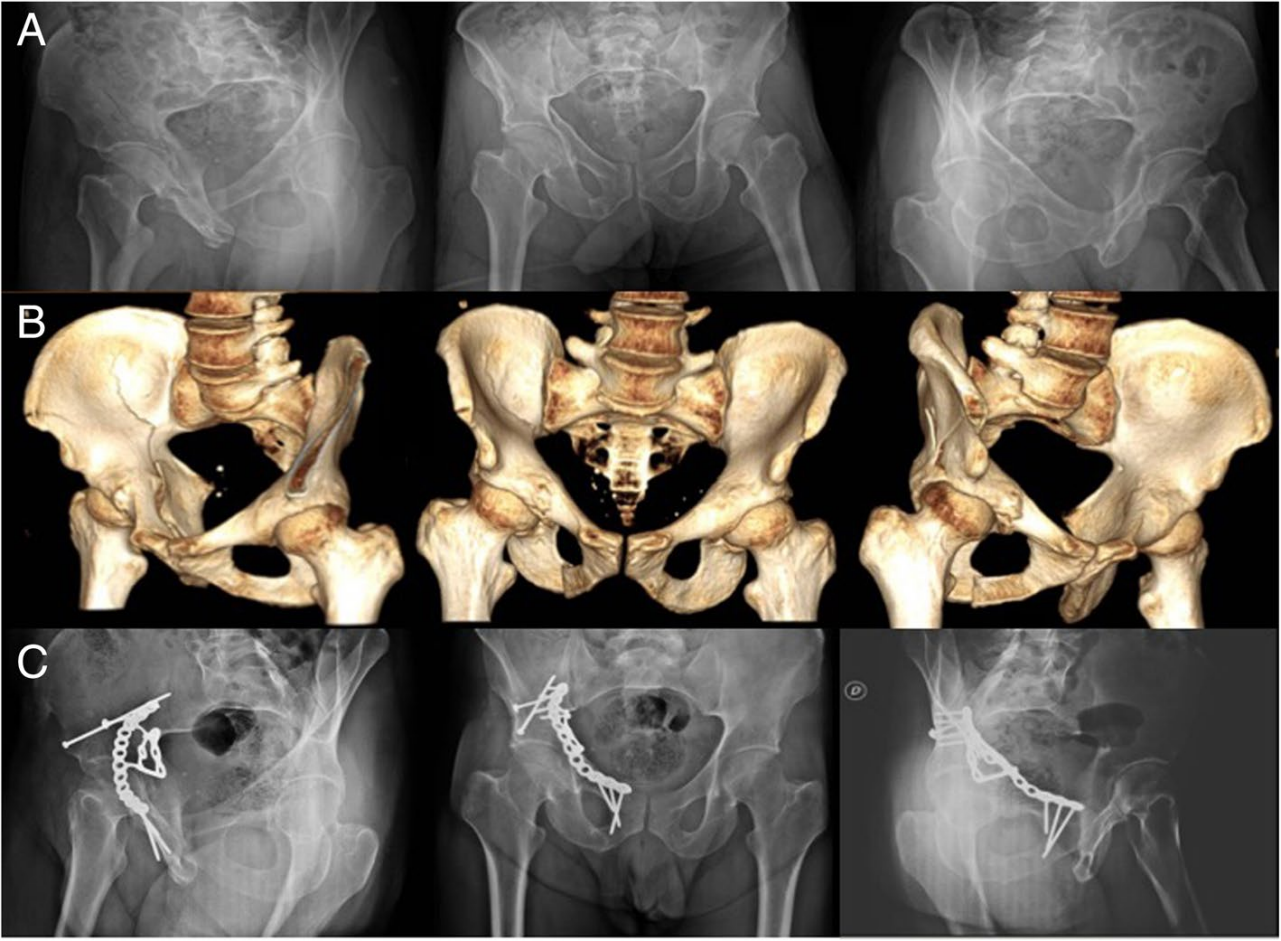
A case of a patient with both columns fracture pattern according to the Judet and Letournel classification of the right acetabulum. It was pre-operative examined with anteroposterior pelvis X-ray view and Judet X-ray views (obturator oblique view and iliac oblique view) (A), and thin-slice CT with 3D reconstructions (B). One-year radiographic follow-up with anteroposterior pelvis X-ray view and Judet X-ray views (C). Internal fixation was performed using the AIP approach and the QLS suprapectineal plate. Also, were used a percutaneous screw inserted with the cannulae of the pelvis pro system and an LC2 screw in the supraacetabular corridor
